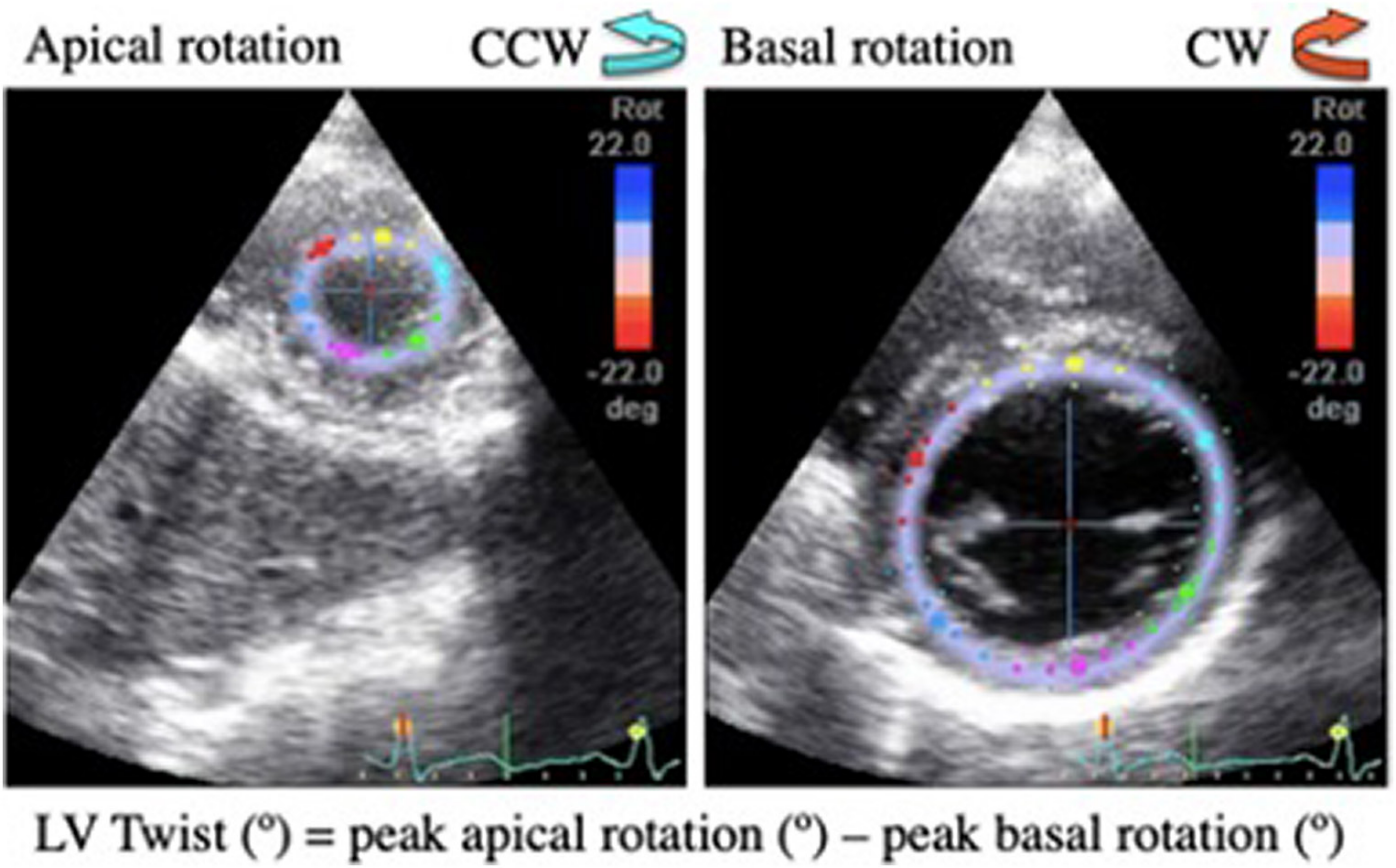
Speckle‐tracking echocardiography analysis of PSAX‐AP and PSAX‐MV images and the subsequent calculation required to obtain LV twist
